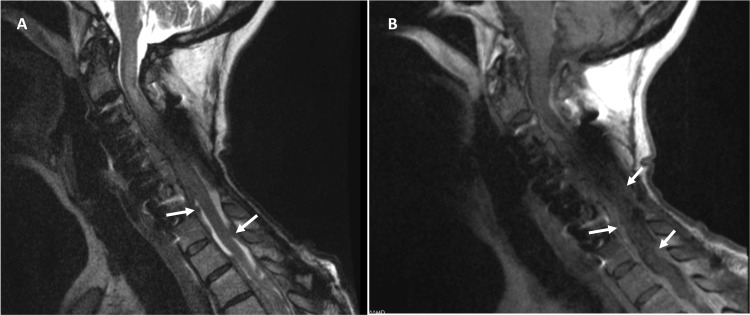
Initial MRI Initial MRI on presentation, with T2-weighted sequence (A) demonstrating cord edema in the upper cervical spinal cord extending from near the cervicomedullary junction inferiorly to roughly the C7 level. Also visualized is myelomalacia distally in the upper thoracic spine likely secondary to circumferential compression from the pachymeningeal pathology. Extramedullary nodular lesions (indicated by arrows) are clearly noted associated with the dura mater. Following contrast administration, these lesions appear to be homogeneously enhancing (B).
